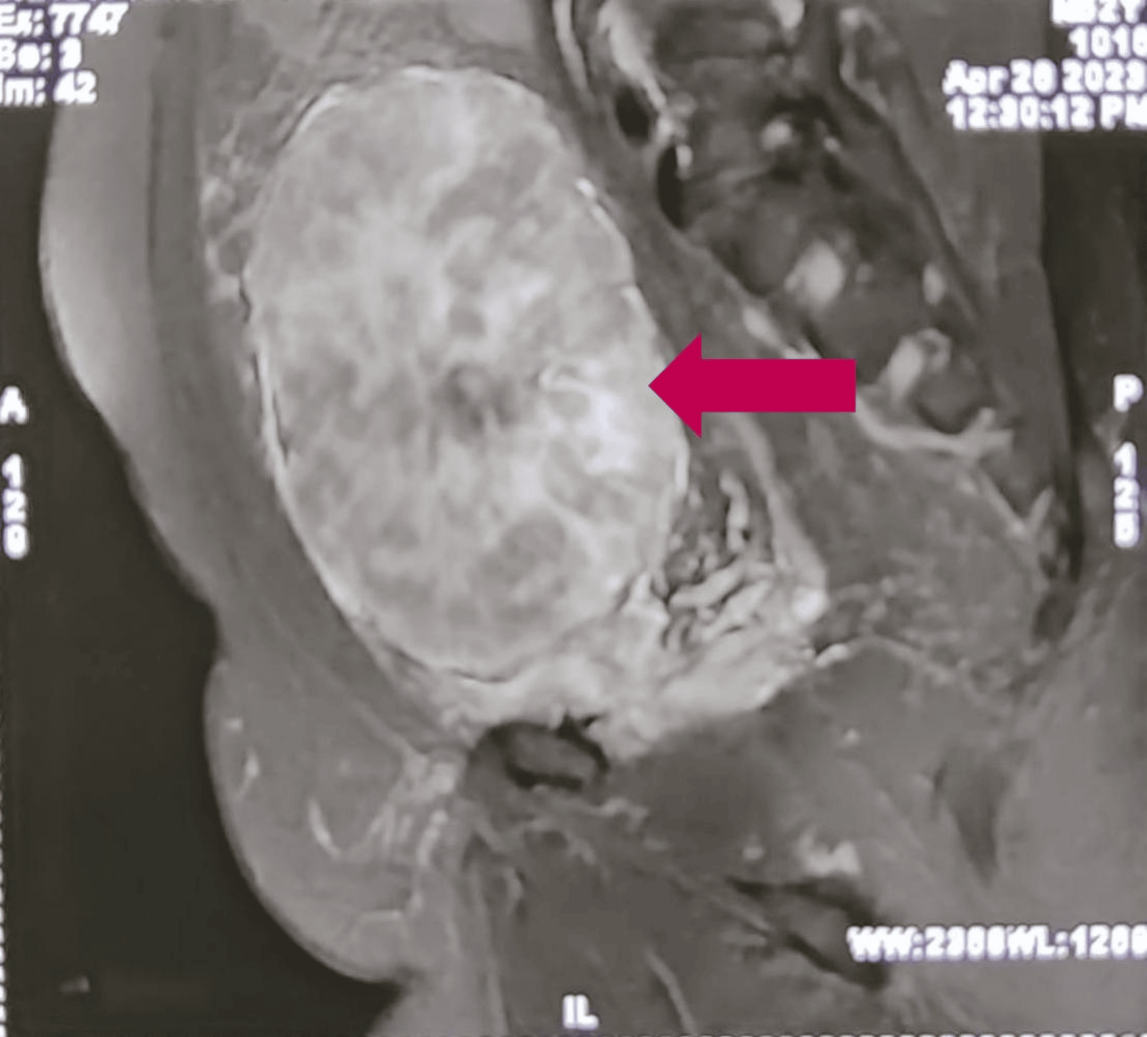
Sagittal section of the MRI abdominal scan showing the tumor (red arrow) arising possibly from the posterior rectus sheath, located in the preperitoneal space MRI: magnetic resonance imaging
